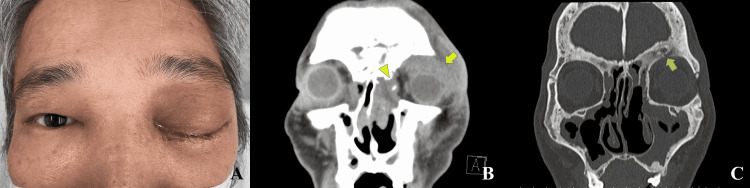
Case presentation (A) A face photo was taken at the first examination showing upper and lower eyelids swelling, proptosis, and chemosis on the left side. (B, C) Coronal computed tomographic images showing orbital abscess (yellow arrow), maxillary and ethmoidal sinusitis, and dacryocystitis (yellow arrowhead). Ground-glass appearance is seen in the frontal, maxillary, and ethmoid bones, and most space of the frontal sinus is obliterated. Cystic lesions are seen in the frontal and maxillary bones, and one cyst opens toward the superior orbit (green arrow).
